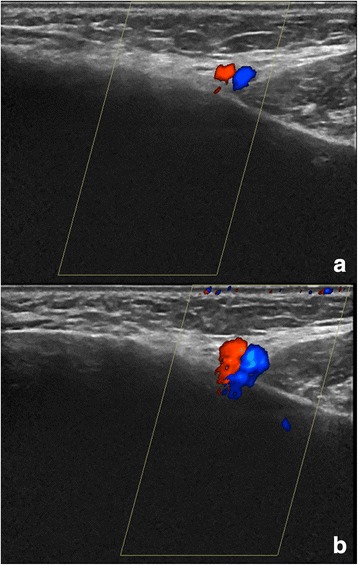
Blooming in a facial artery and vein. Blooming is gain-dependent and lowering the Doppler gain will minimize blooming artefacts (*top*), while increasing gain also increases blooming (*bottom*)
